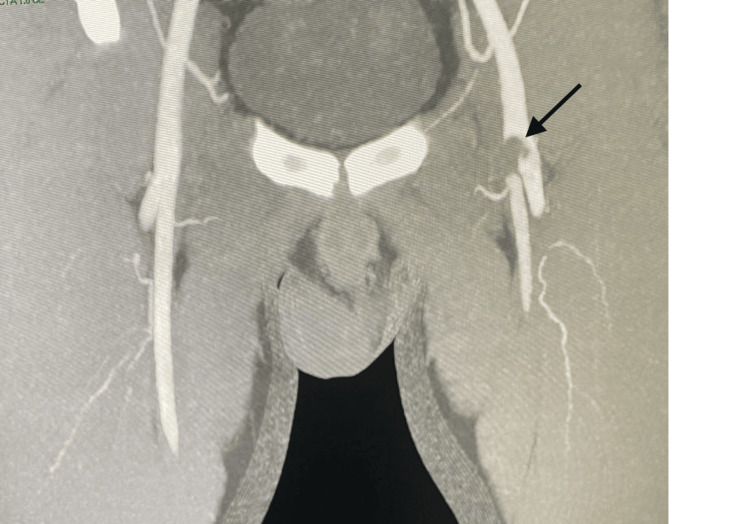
CT angiogram CT, computed tomography
